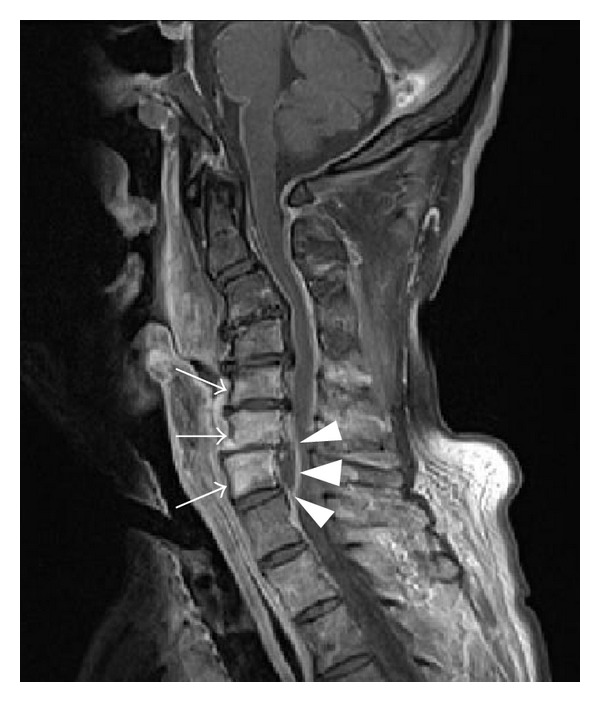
Magnetic resonance T1-weighted, contrast-enhanced image at the time of the third admission. Arrows indicate high signal on vertebrae C4-C7. Arrow heads indicate an epidural abscess.
